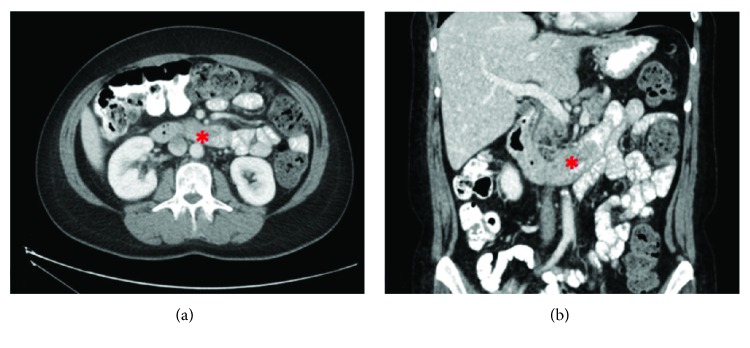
Axial (a) and coronal (b) computed tomography images of the duodenal GP, appearing as a hypodense lesion, shown to arise from the second/third portion of the duodenum and abut the uncinate process of the pancreas. Tumor is marked by an asterisk.
